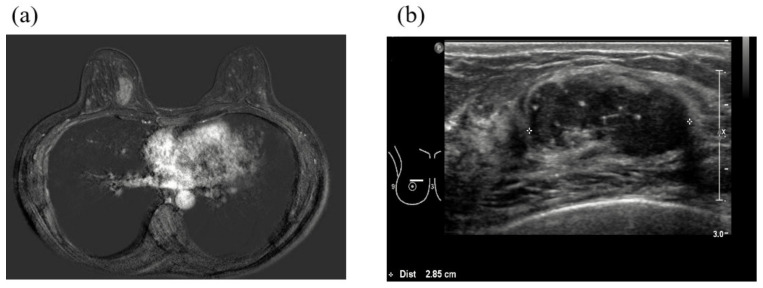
Imaging of patient No. 1 at the first diagnosis of breast cancer in January 2016. (a) Breast magnetic resonance imaging (MRI) showed a 2.4 cm lesion in the right breast. (b) Breast ultrasound image showed a hypoechoic solid lesion in the right breast.
